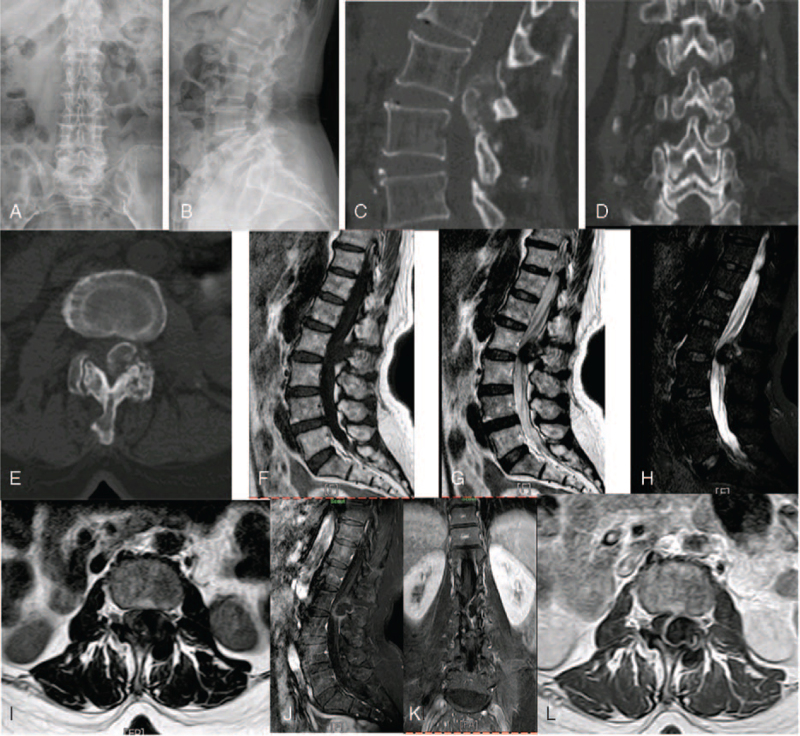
Preoperative images: (A, B) Lumbar anteroposterior and lateral radiographs: mass like calcification shadow can be seen at the left facet joint of lumbar 2–3; (C—E) CT plain scan of lumbar vertebrae: hyperosteogeny at the edge of lumbar vertebrae, bone destruction of the left superior and inferior articular process of lumbar vertebrae at lumbar 2 and 3 levels and slightly high density around the vertebral body, the focus protruded into the spinal canal, the size was about 2.7 x 1.8 x 4.0 cm, the shape was irregular, the edge was calcified, the internal density was uneven and multiple dotted high-density shadows were seen; (F—L) MRI enhancement of lumbar vertebrae: irregular cystic solid masses were seen outside the spinal canal on the left side of lumbar 2 and 3 vertebrae, especially solid components, bone destruction of the superior and inferior articular process and encapsulated calcification around articular process were seen. The enhanced scan showed solid part and septal enhancement, compression of the adjacent spinal cord, and the lesion grew laterally through the left intervertebral foramen of lumbar 2–3 and 3–4.
